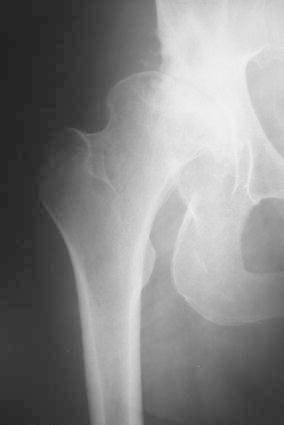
Preoperative radiographic aspect in anteroposterior view of a 59 year-old female with Crowe grade 1 congenital hip dysplasia
